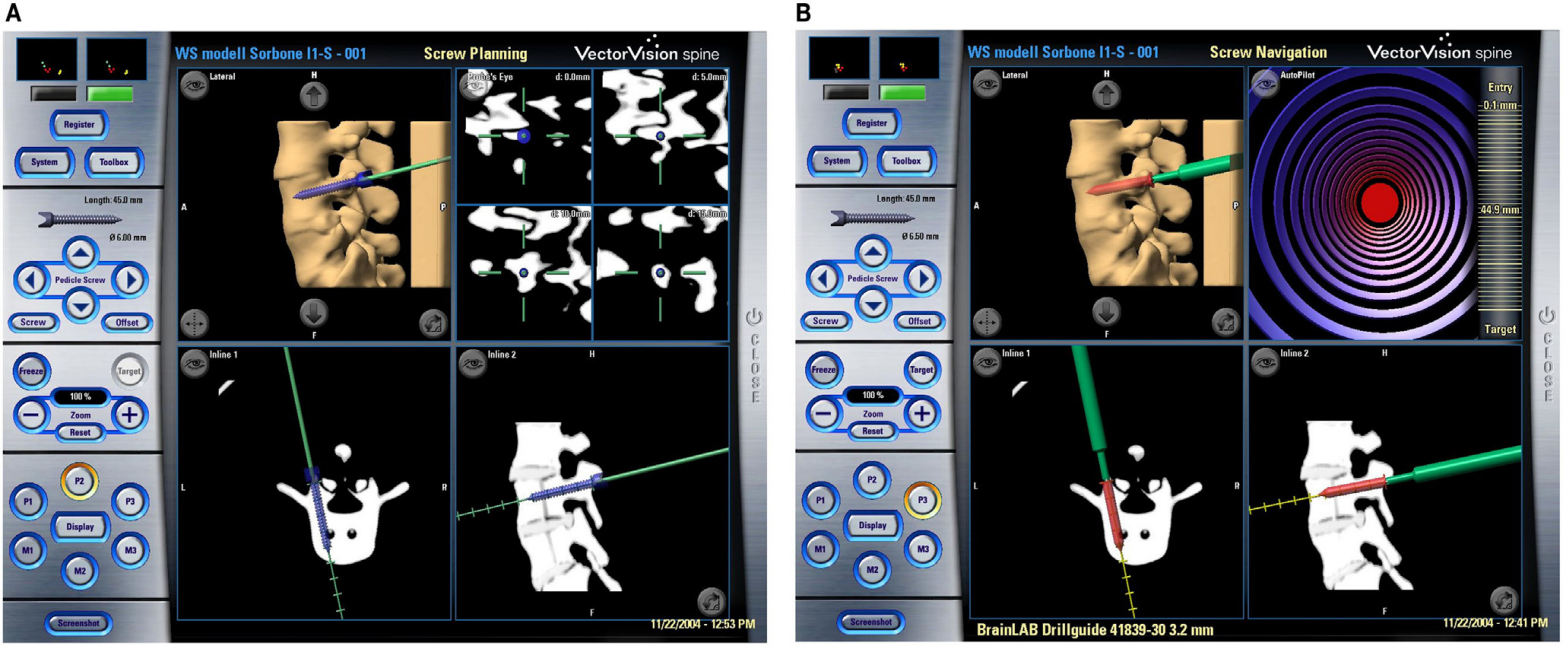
Example of CT-based navigational feedback. These screenshots show a CT-based CAOS system during pre-operative planning (A) and intra-operative navigation (B) of pedicle screw placement (Courtesy of BrainLAB AG, Munich, Germany).
